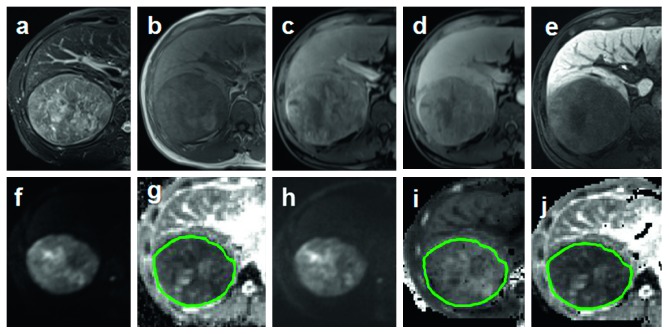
ROI was drawn on the level of maximum transactional diameter of HCC (green circle). (a, b) Axial fat suppress T2WI and T1WI. (c–e) Dynamic enhancement imaging including artery phase, portal phase, and hepatobiliary phase (HBP). (f, g) Axial liver DWI (*b* = 800 s/mm^2^) and ADC maps. (h–j) Axial liver DKI (*b* = 2000 s/mm^2^) and DKI-derived parameters *K* and *D* maps.
